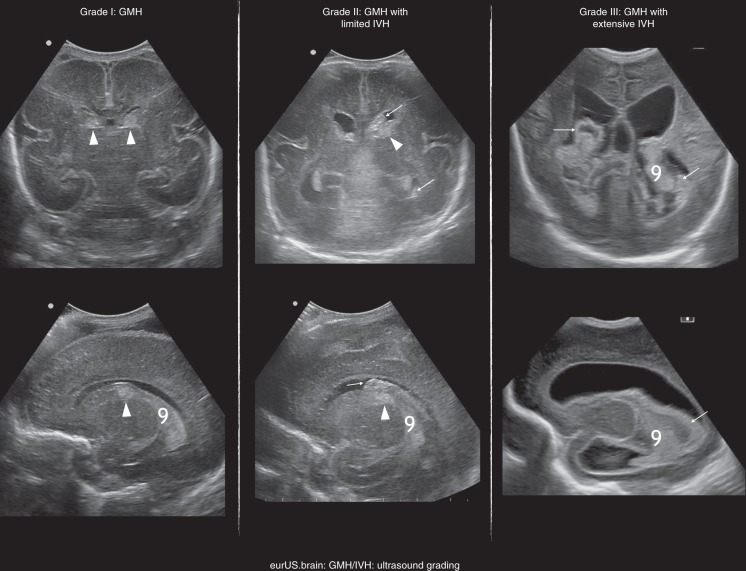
GMH/IVH: ultrasound grading. CUS grading of GMH/IVH; arrowheads point to GMH, arrows to the presence of clot in the ventricle cavity; asterisk is choroid plexus.
